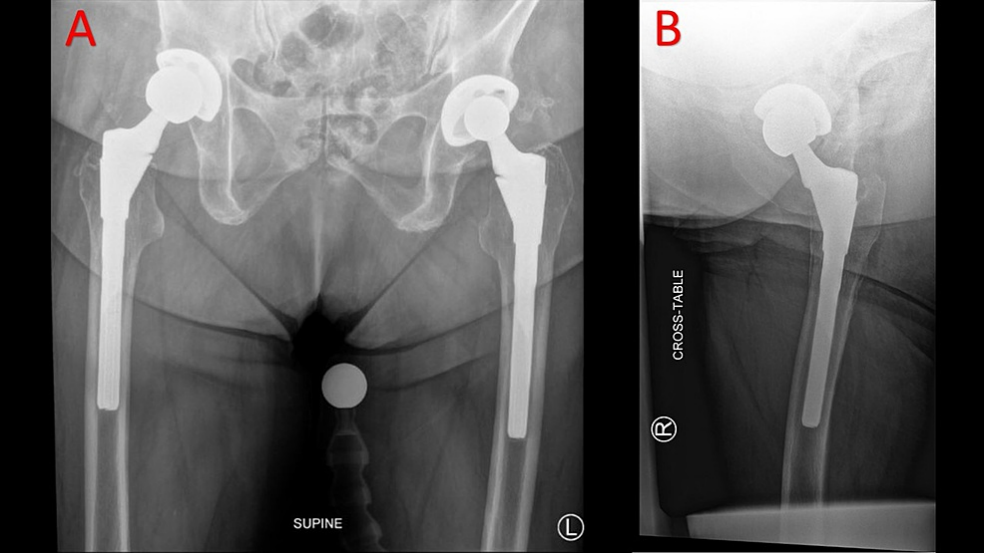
Preoperative radiographs Panel A: Preoperative anterior-posterior pelvis radiograph demonstrating minimal anteversion of the right acetabular component. Panel B: Preoperative cross-table lateral radiograph of the right hip
